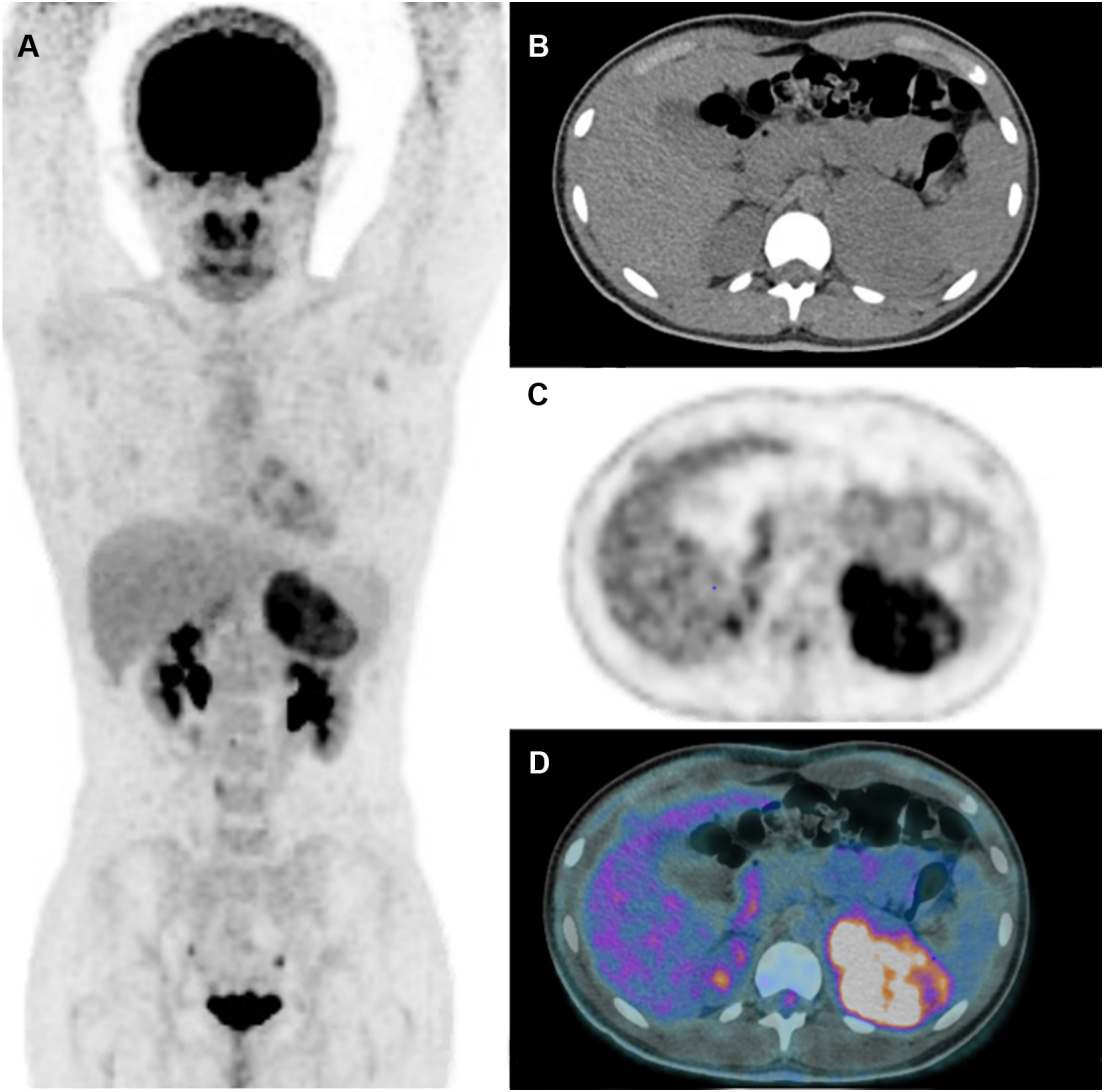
Adrenocortical carcinoma. 13-year-old girl with hyperandrogenism (secondary amenorrhea, hirsutism). Large adrenal mass on US and CT scan. [^18^F]FDG PET shows uptake in a left adrenal mass without metastasis. After surgery, pathological analysis confirmed the diagnosis of ACC with Wieneke score 3 (55), Ki-67 index 5.3% and p53-negative status. Complete remission 5 years after surgery. Maximum intensity projection image (A) Transverse CT (B), PET (C), PET/CT fusion image (D). (Courtesy of Drs. Catherine Ansquer and Morgane Cleirec from Nantes University Hospital/Hôtel-Dieu).
